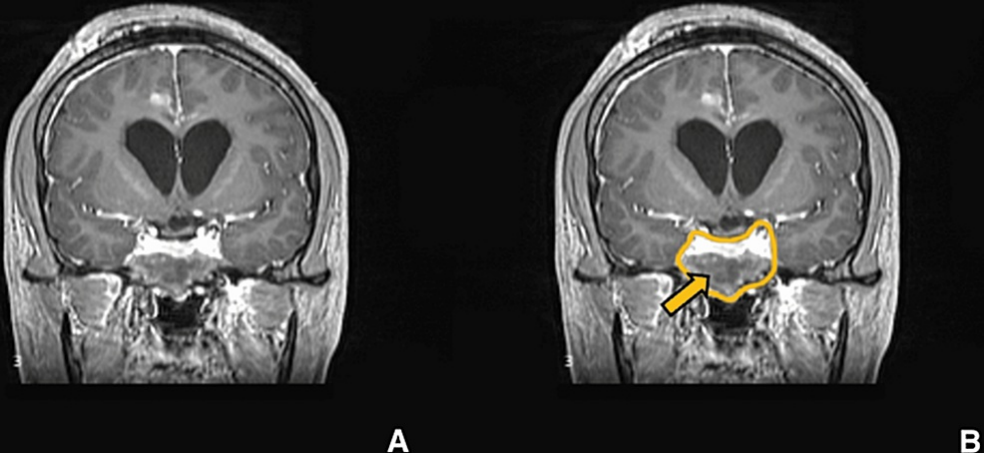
Postoperative imaging for patient 1. Contrast-enhanced T1-weighted coronal image showing significant reduction in size of the lesion. (A) Without ROI and (B) with ROI. The yellow arrow is pointing to the hypointense region where the mass has been reduced.
